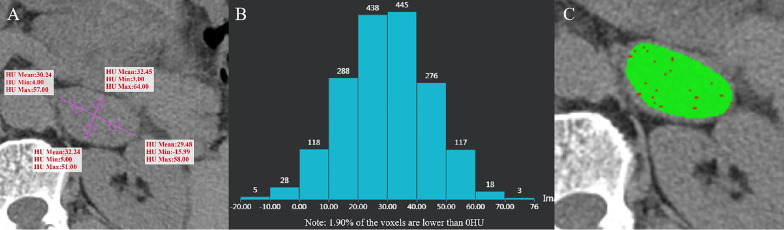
Ganglioneuroma of the left adrenal gland in a 55-year-old man. AV_mean_ = 31.103 HU, AV_min_ = -0.75 HU (A), and CTH negative pixel =1.9% (B). The CT value in the red area is ≤10 HU, and the CT value in the green area is > 10 HU (C).
